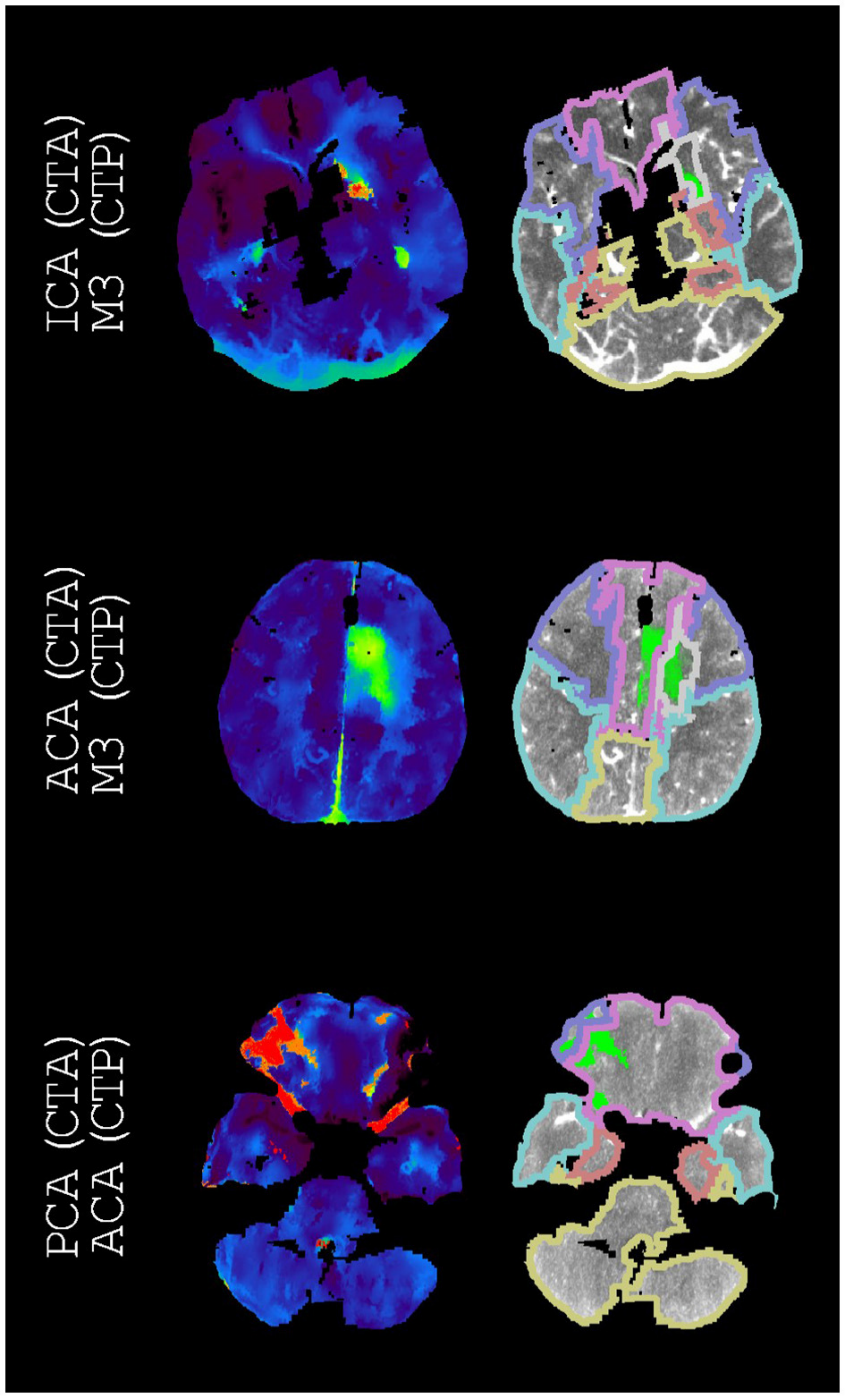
Examples of annotations (CTA) and indications (CTP) that do not match arteries other than the middle cerebral artery. On the left, time-to-peak perfusion maps are shown. On the right, the hypoperfused regions and the downstream regions (according to Figure 1) are shown. For each example, we only regarded the M3 downstream region with the highest intersection-over-union. If displayed, these M3 downstream regions are the frontal white matter and the frontal white matter (from top to bottom). The second-best indications were M2, ACA, and M2 (from top to bottom).
